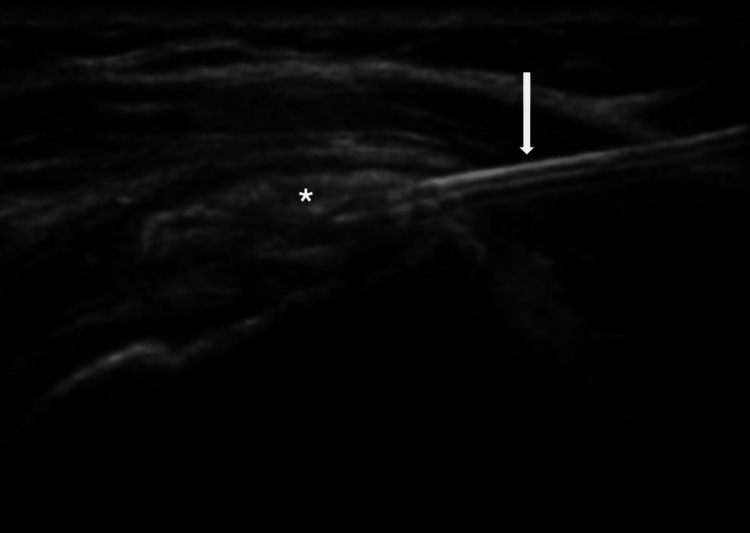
Ultrasound image of the needle (arrow) entering the calcification (asterisk) This is an original image.
